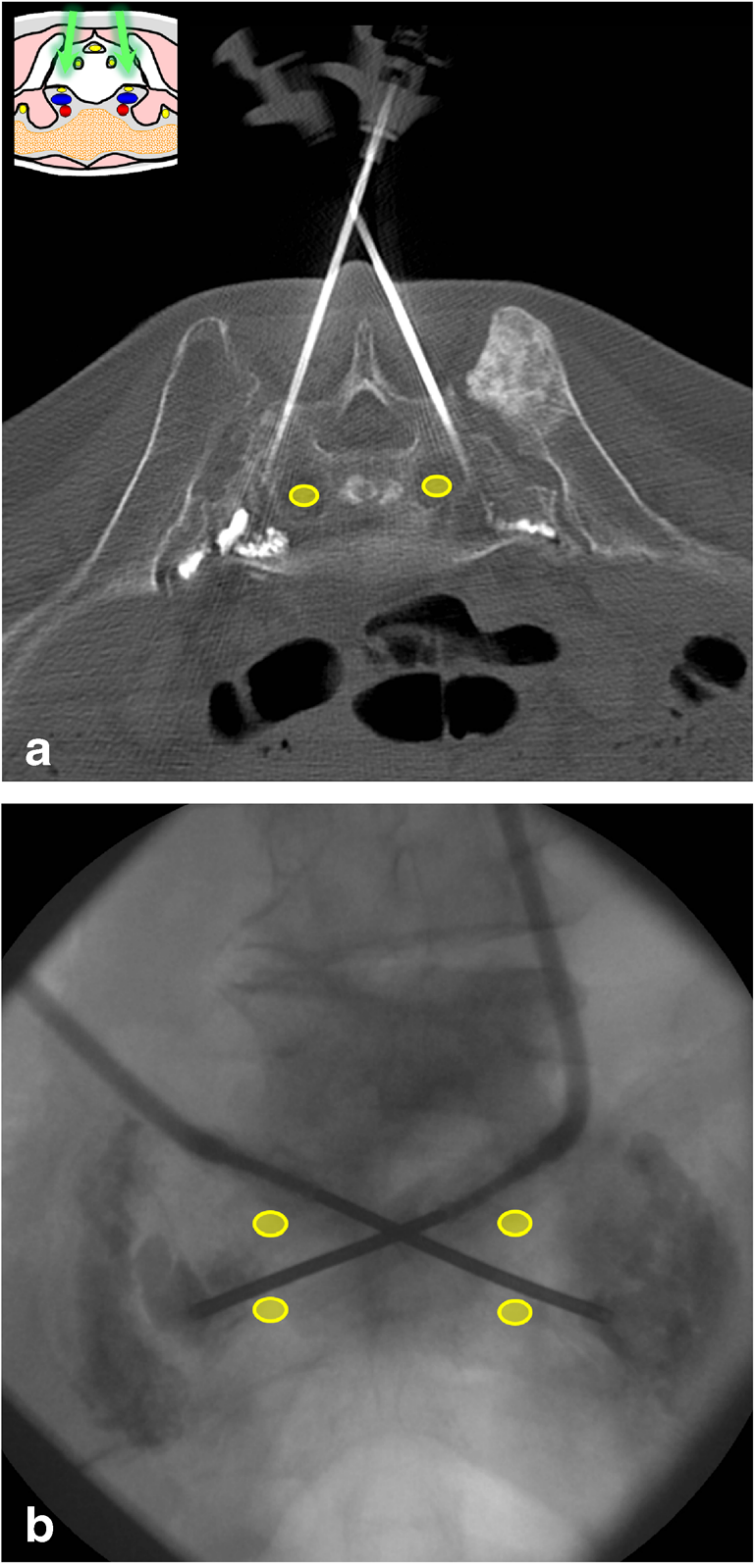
A 70-year-old woman with metastatic lung cancer and sacral insufficiency fractures. a Axial CT shows the 13-G bone needles inserted in the sacral wings and the beginning of the cement injection. b PA fluoroscopy view is essential to appreciate the real-time cement distribution and confirms the filling of the fracture lines on both sides. Special attention should be paid for sacral foramina during this procedure
